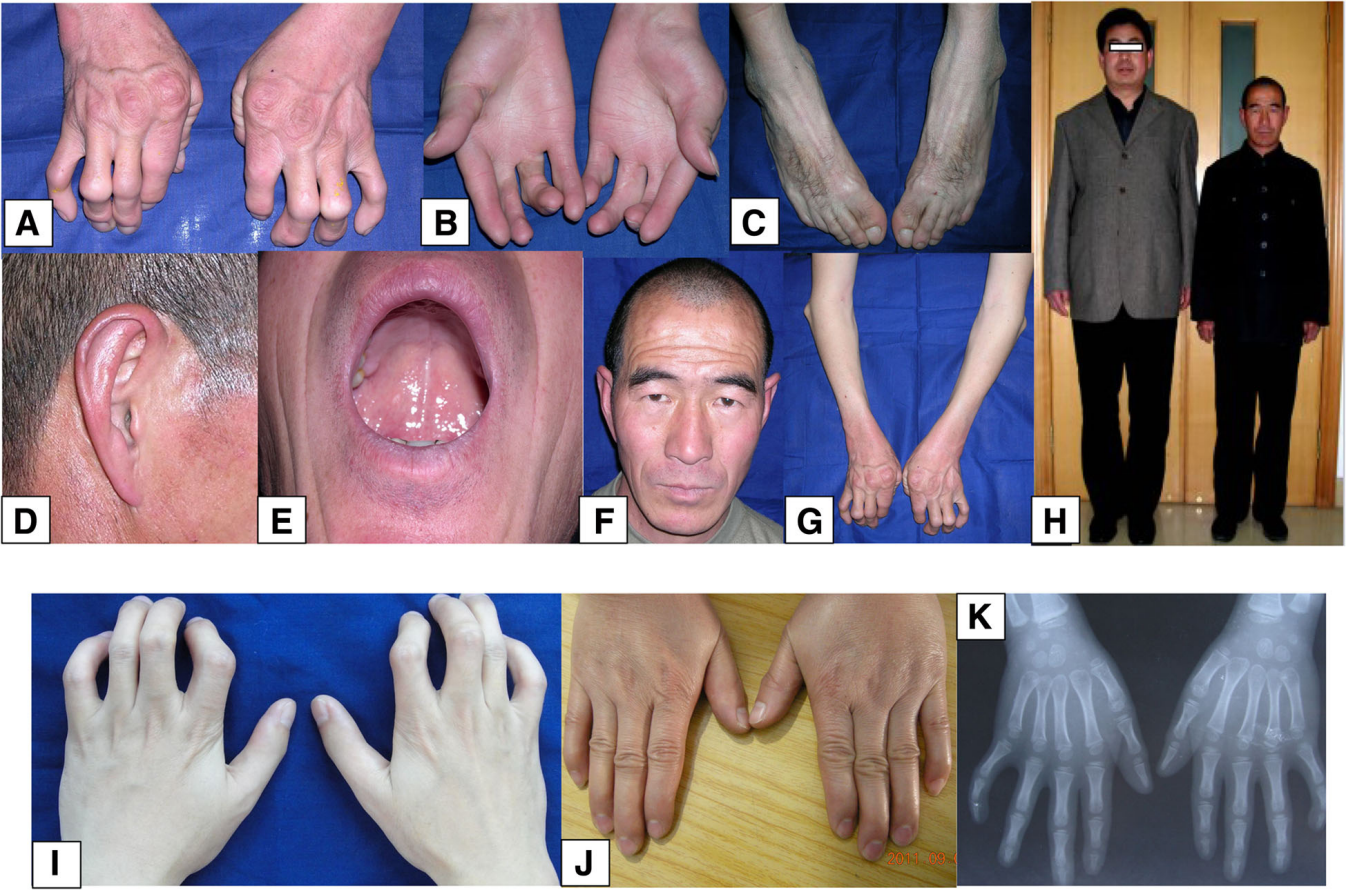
Clinical features of patients in Family 1 with DA2B (a-h) and Family 2 with mild DA (i-k). a Ulnar deviation and contractures in patient II5. b Camptodactyly and adducted thumbs in patient III1. c Flexed toes and talipes equinovarus in patient II5. d Dysplastic ear and attached ear lobes in patient II5. e Small mouth with limited opening in patient II5. f Downward-slanting palpebral fissures, a small chin, and deep folds in the nasolabial area and forehead in patient II5. g Flexion contracture yielding stiff elbows in patient II5. h Short stature of patient II5 (unaffected II6 [left], 178 cm; patient II5 [right], 156.5 cm). i Camptodactyly and ulnar deviation in patient II5. j Mild contractures of the fingers in patient II2. k X-ray findings of patient III1 in 5 years old indicate camptodactyly and ulnar deviation
